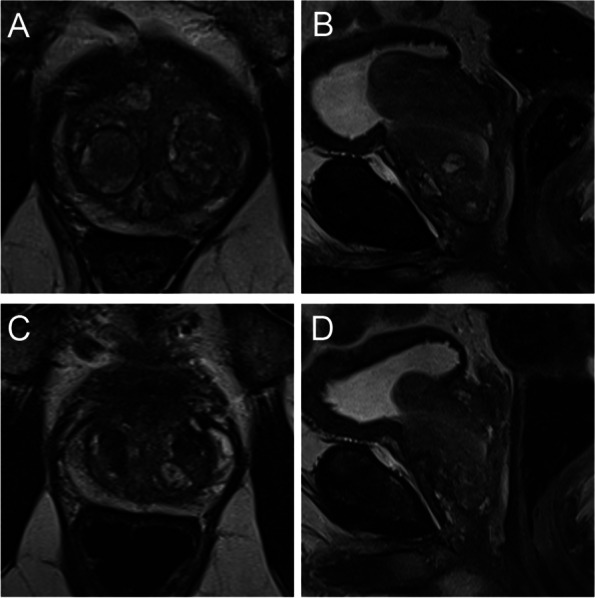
Wasserman type 2, modified BPH classification 2 (retrourethral); A T2-weighted axial baseline B T2-weighted sagittal baseline; retrourethral dominant hyperplasia with pedunculated nodules; C T2-weighted axial follow-up D T2-weighted sagittal follow-up. Volume reduction of 95 ml to 51 ml (46%); IPSS 12 to 6 (50%)
